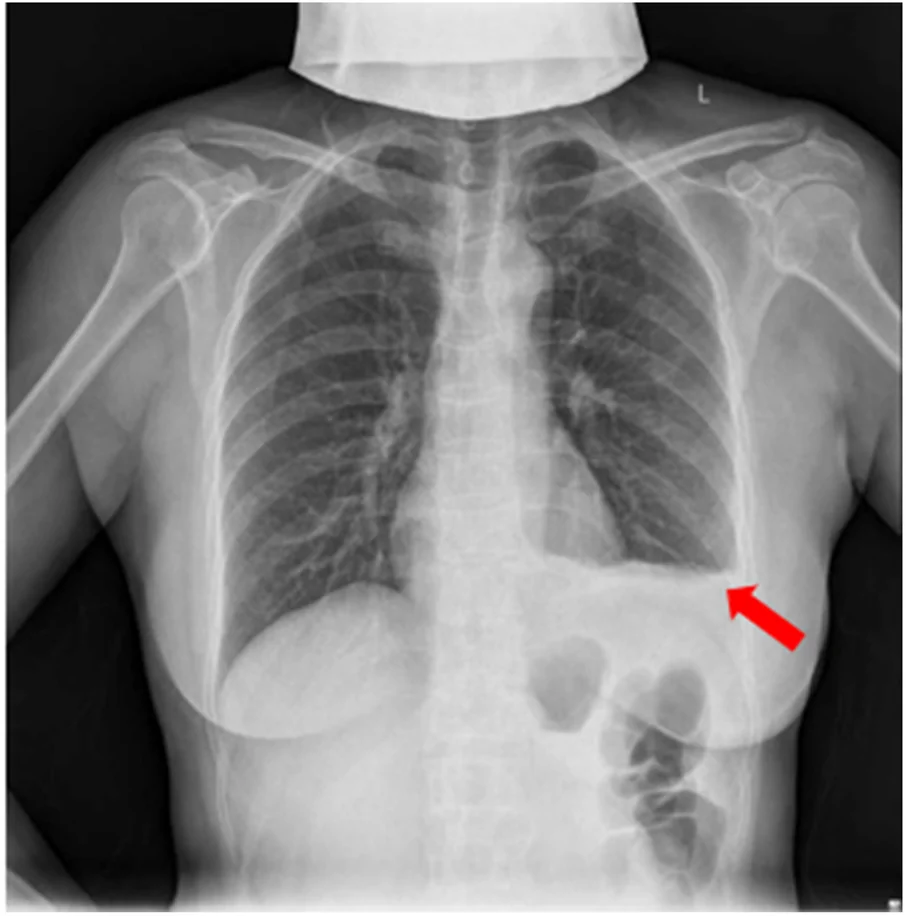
Chest radiography on admission showing reduced left lung volume and elevation of the left hemidiaphragm.
